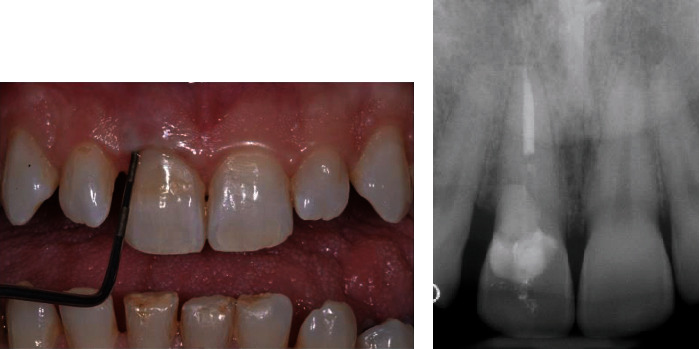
(a) Probing and postoperative clinical condition and (b) X-ray at the 6-year follow-up.
